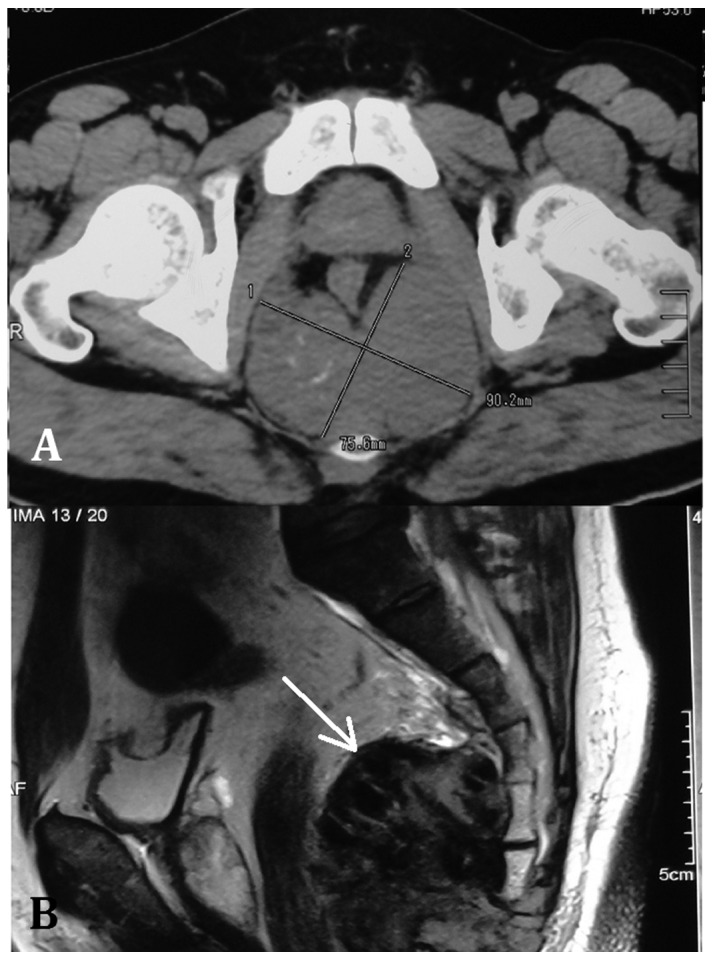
Case one. (A) Transverse and (B) sagittal plane magnetic resonance imaging showing an irregularly shaped mass, 7.5×9.1×9.3 cm in size.
